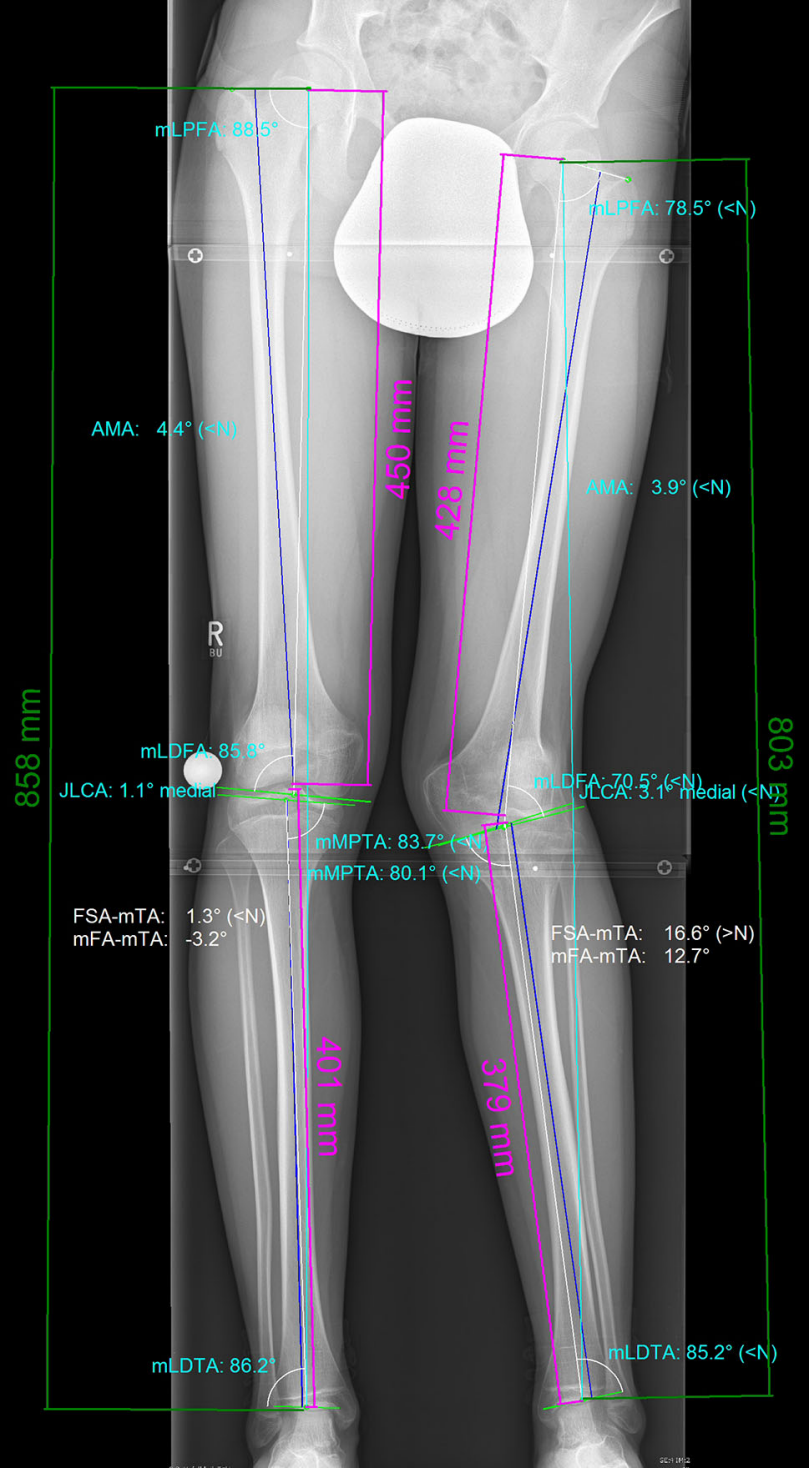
Preoperative full-weight-bearing long-leg radiograph. Analysis of the deformity with the planning software mediCAD (Hectec, Germany), angles according to Dror Paley. Measurements are in Table 1
